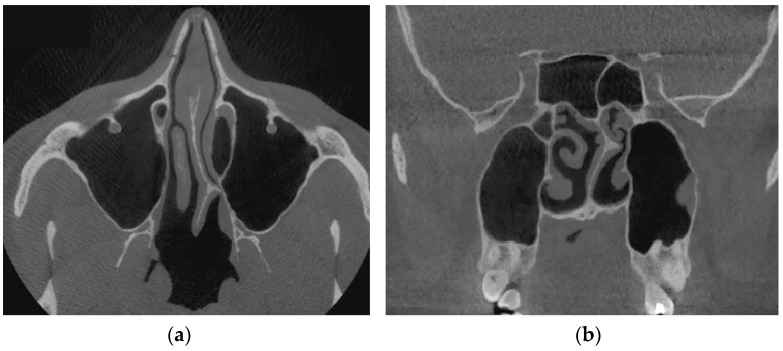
Computed tomography of a patient with curvature of the nasal septum to the left in the posterior part: (a) axial tomographic section; (b) multiplanar reconstruction in frontal projection.
